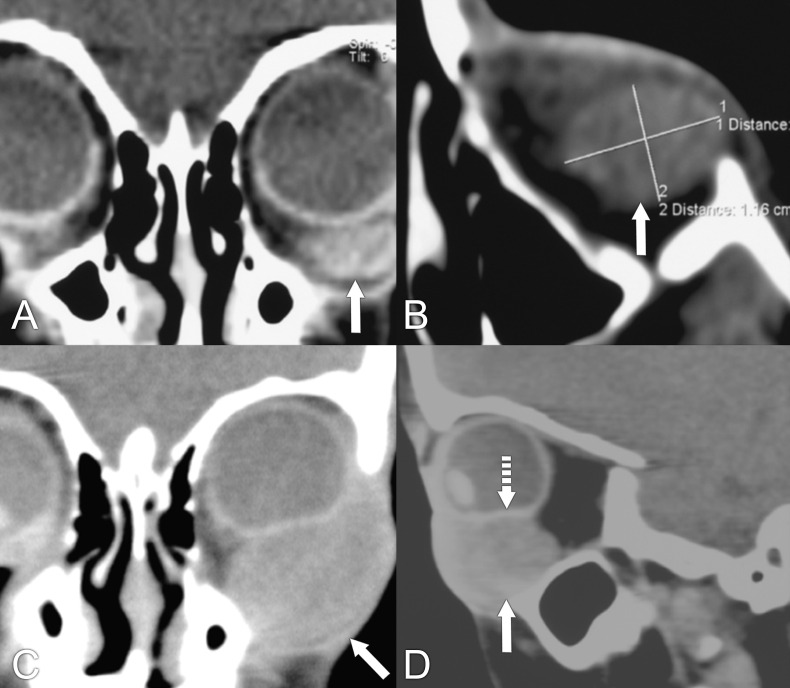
Imaging findings in a 4-year-old boy who presented with an acute onset of proptosis. (A, B) Coronal and sagittal CT with contrast, soft tissue windows acquired in the emergency department in his home country in the Middle East, show an inferior orbital mass (arrows). (C, D) Coronal and sagittal CT with contrast, soft-tissue windows acquired 4 weeks later, show significant enlargement of the mass, which is typical of rhabdomyosarcoma (solid arrows). The coronal image shows that the inferior orbital mass had almost tripled in size in just 4 weeks. The sagittal image shows globe distortion by the mass (dashed arrow). A biopsy of the orbital mass confirmed the diagnosis of embryonal rhabdomyosarcoma.
